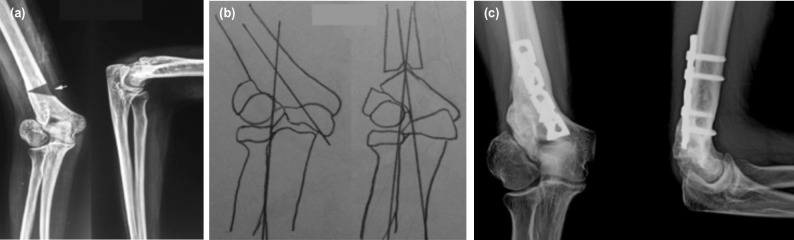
(a) A 18-year-old female patient presented with TUNP secondary to cubitus valgus deformity right side - Pre-operative radiograph showing non-union of the lateral condyle humerus and cubitus valgus deformity (36° valgus). White arrow shows site of planned osteotomy. Black overlaid triangle shows site of planned osteotomy. (b) Milch osteotomy was done to correct the deformity and fixed with plate, without osteosynthesis of the non-union and anterior transposition of ulnar nerve. (c) Six months post-operative radiograph showing union at the osteotomy site and carrying angle corrected to 10° valgus. Excellent results were seen for deformity correction and ulnar nerve symptoms.
